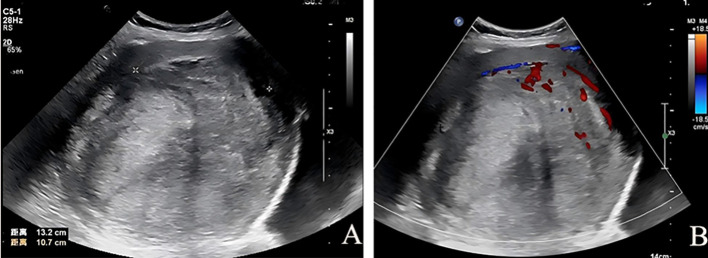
Pelvic ultrasound images. (A) A mixed echogenicity mass measuring 132 mm × 107 mm is observed in the uterus, with a clear boundary and heterogeneous internal echogenicity. (B) Color Doppler Flow Imaging (CDFI) shows scant intratumoral blood flow and a semi-annular blood flow signal surrounding the mass.
